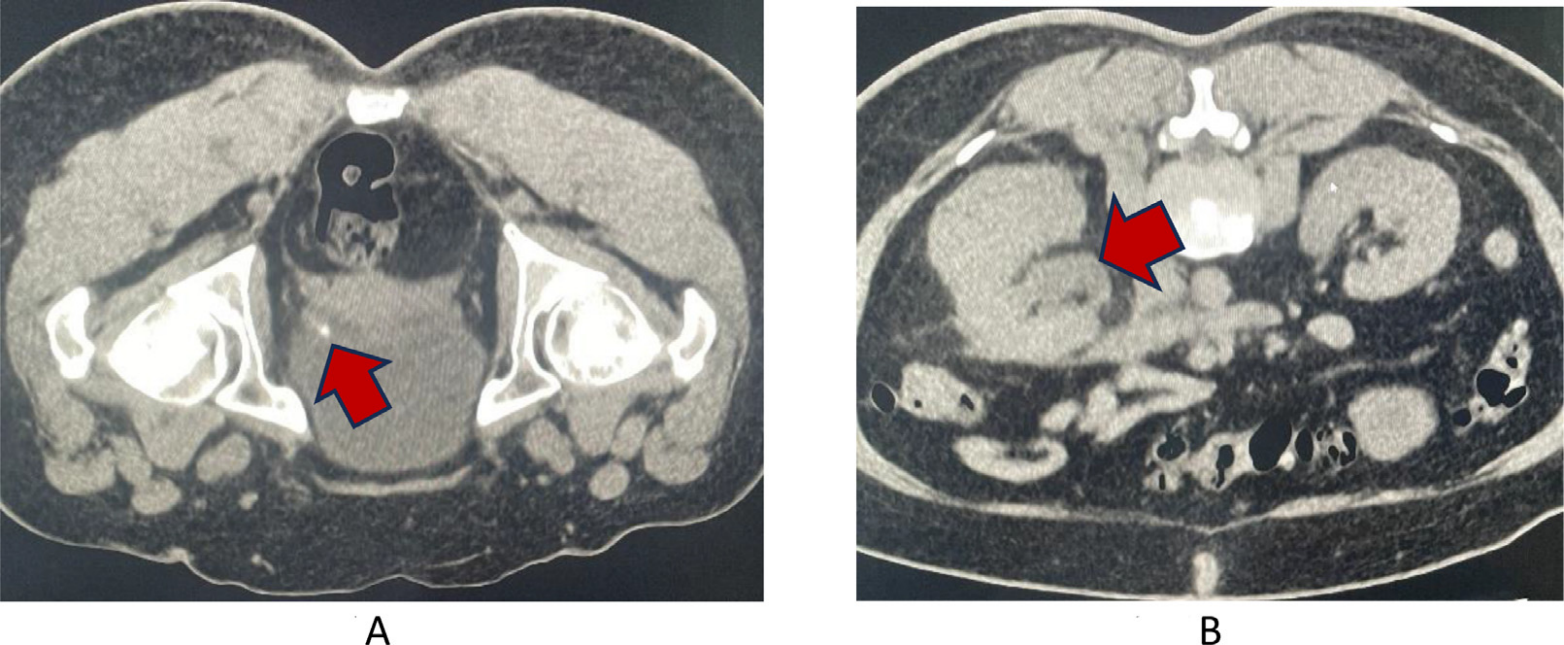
Imaging modality: noncontrast enhanced computed tomography. Findings: (A) a 4 mm obstructing stone at the level of the right VUJ (red arrow). (B) Proximal hydronephrosis and hydroureter as well as some mild enhancement of the right renal pelvis and distal ureter (red arrow).
